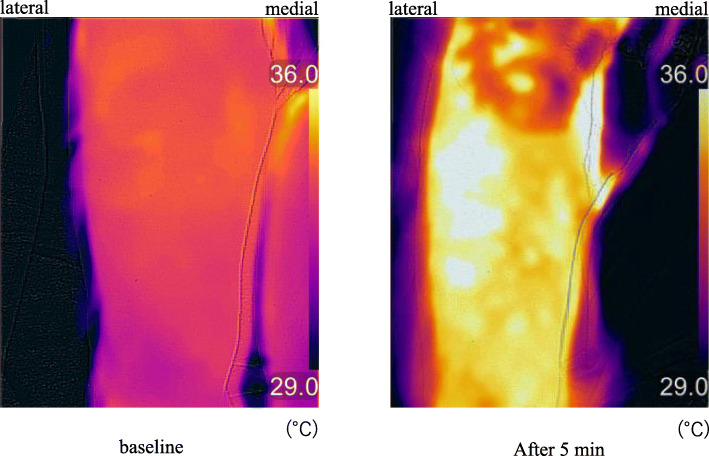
Thermographic images acquired before and 5 min after ultrasound-guided supra-inguinal fascia iliaca block. Thermography predominantly in the lateral femoral nerve site in patient no. 1 resulted in an increase in skin temperature in the middle of lateral femoral site was increased from 33.6 to 35.8°C by nerve block
